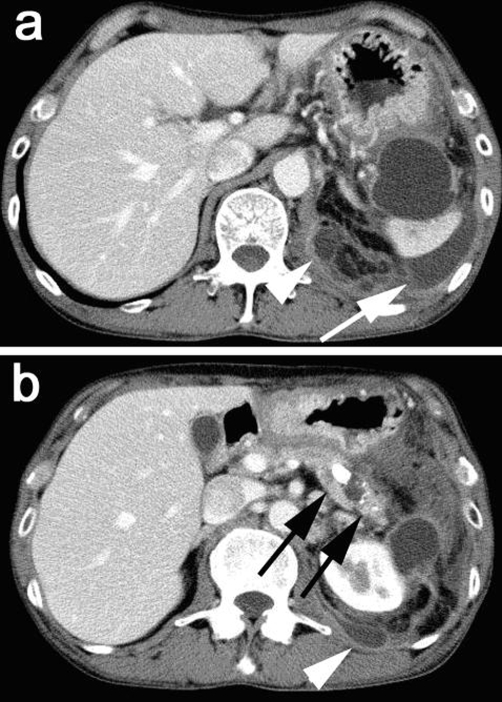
An abdominal CT scan at the 1st consultation showed many stones in the main pancreatic duct (black arrows in b) and a cystic lesion, 4 cm diameter, located in the pancreatic tail and surrounded by the stomach, the spleen and the left kidney. The cystic lesion extended to the dorsal side of the left kidney (white arrowhead in b) and there was small amount of fluid collection around the spleen, which was considered to be caused by acute inflammation on chronic pancreatitis (white arrow in a).
